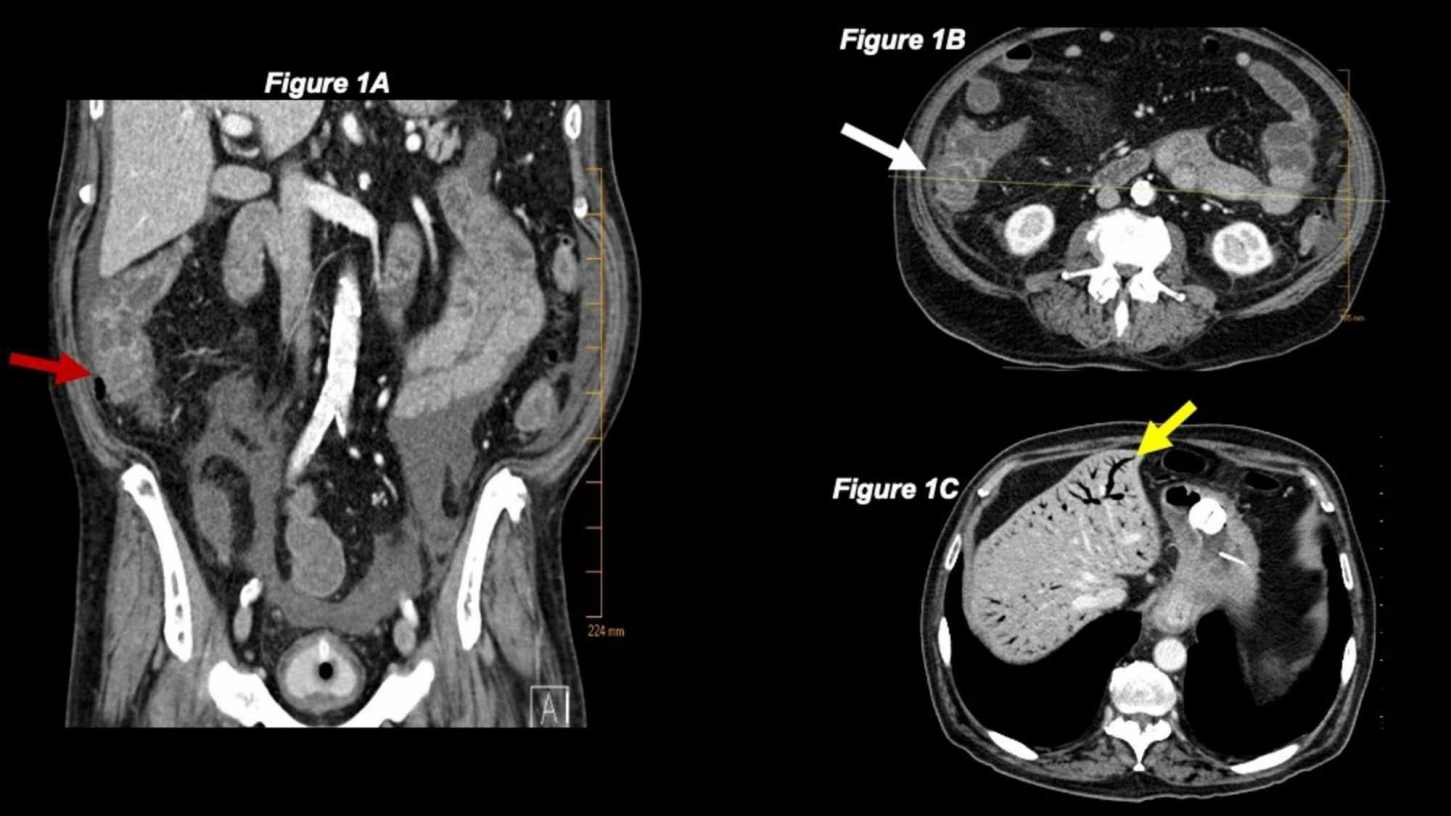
CT of the abdomen revealing the site of neutropenic enterocolitis and portal venous gas (A) Coronal view of CT abdomen showing the site of neutropenic enterocolitis and gas in paracolic gutter (red arrow). (B) Transverse view of CT abdomen showing the site of neutropenic enterocolitis (white arrow). (C) Transverse view of CT abdomen showing significant portal venous gas that eventually spontaneously resolved within hours on repeat CT abdomen (yellow arrow).
